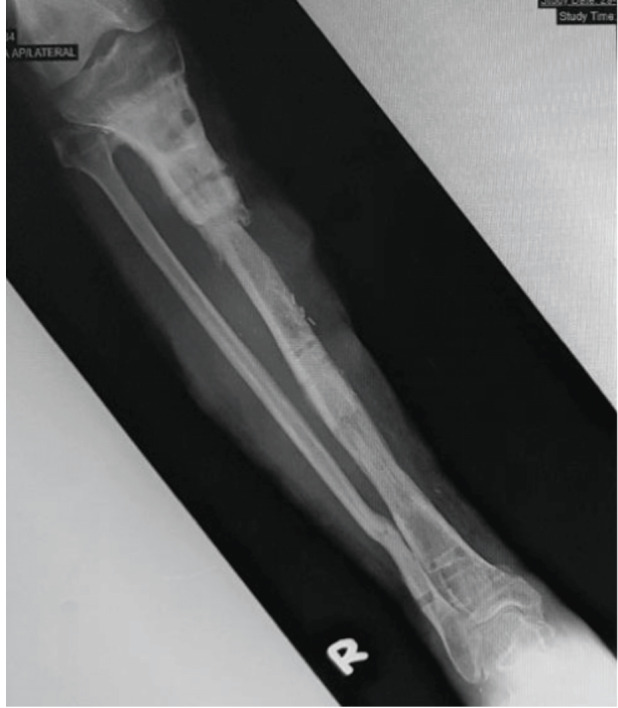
Radiograph showing bone defect consolidation 12 months after Ilizarov external fixator application.
